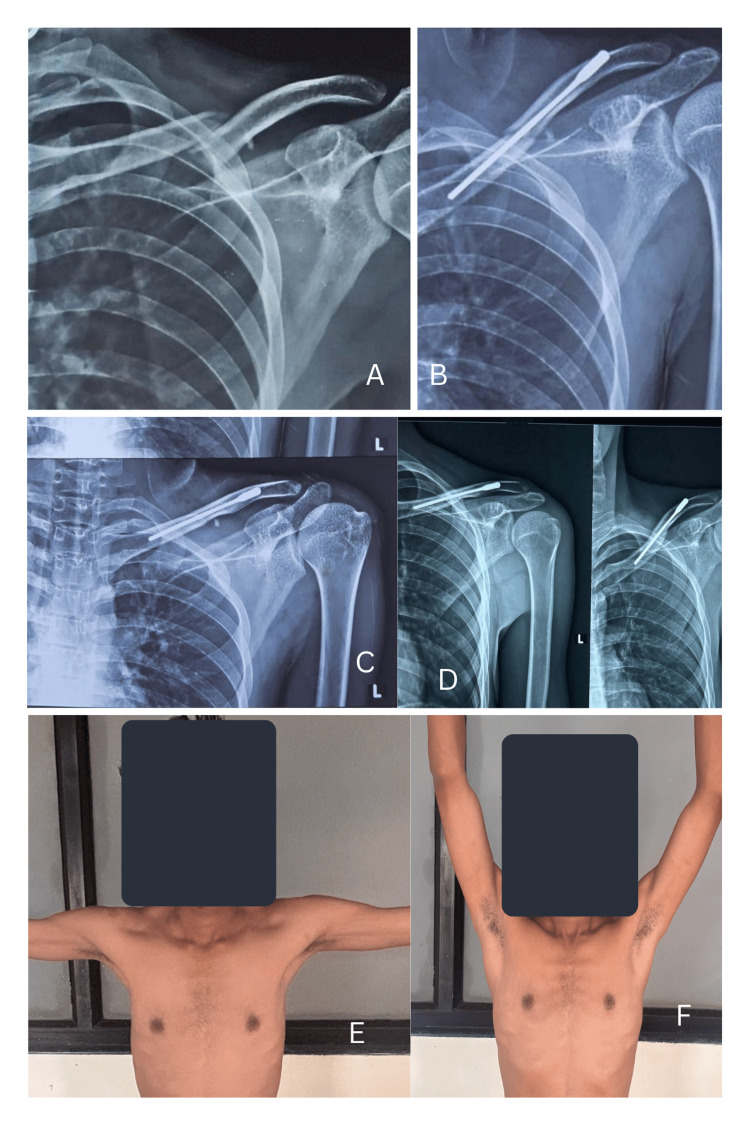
A 30-year-old male patient with a middle-third clavicle fracture treated with a screw nail. (A) Preoperative radiograph showing an Allman Type 1 displaced clavicle fracture; (B) Immediate postoperative radiograph; (C) Radiograph at 6 months; (D) Radiograph at 1 year postoperatively; (E, F) Clinical images showing complete shoulder range of motion.
